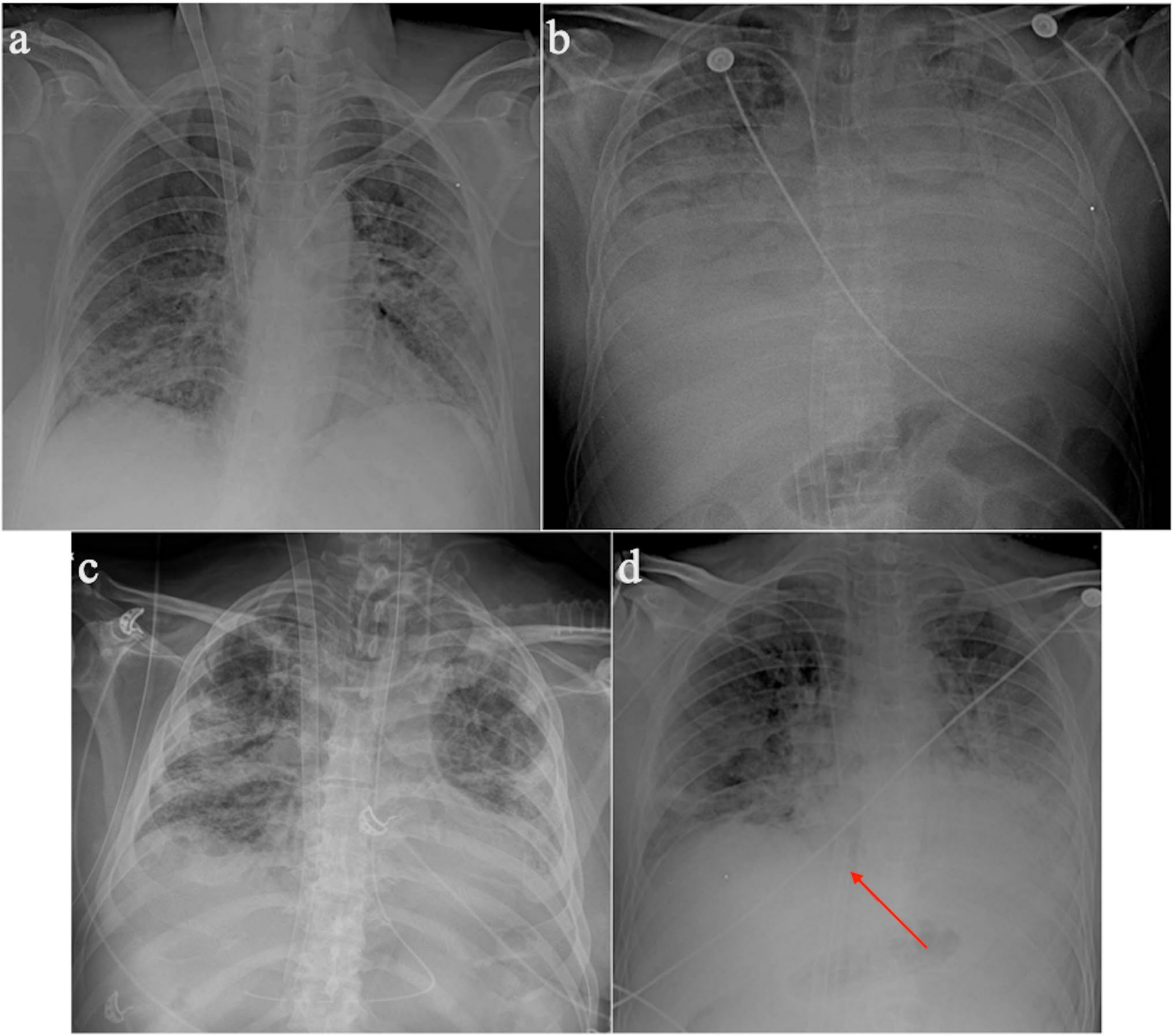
Chest radiographs (antero-posterior view) showing a correct placement of VV-ECMO, b correct placement of femorofemoral ECMO, c correct placement of double lumen ECMO, d incorrect placement of femorofemoral ECMO. The tip of the drainage cannula (arrow) is too close to the tip of the return cannula
